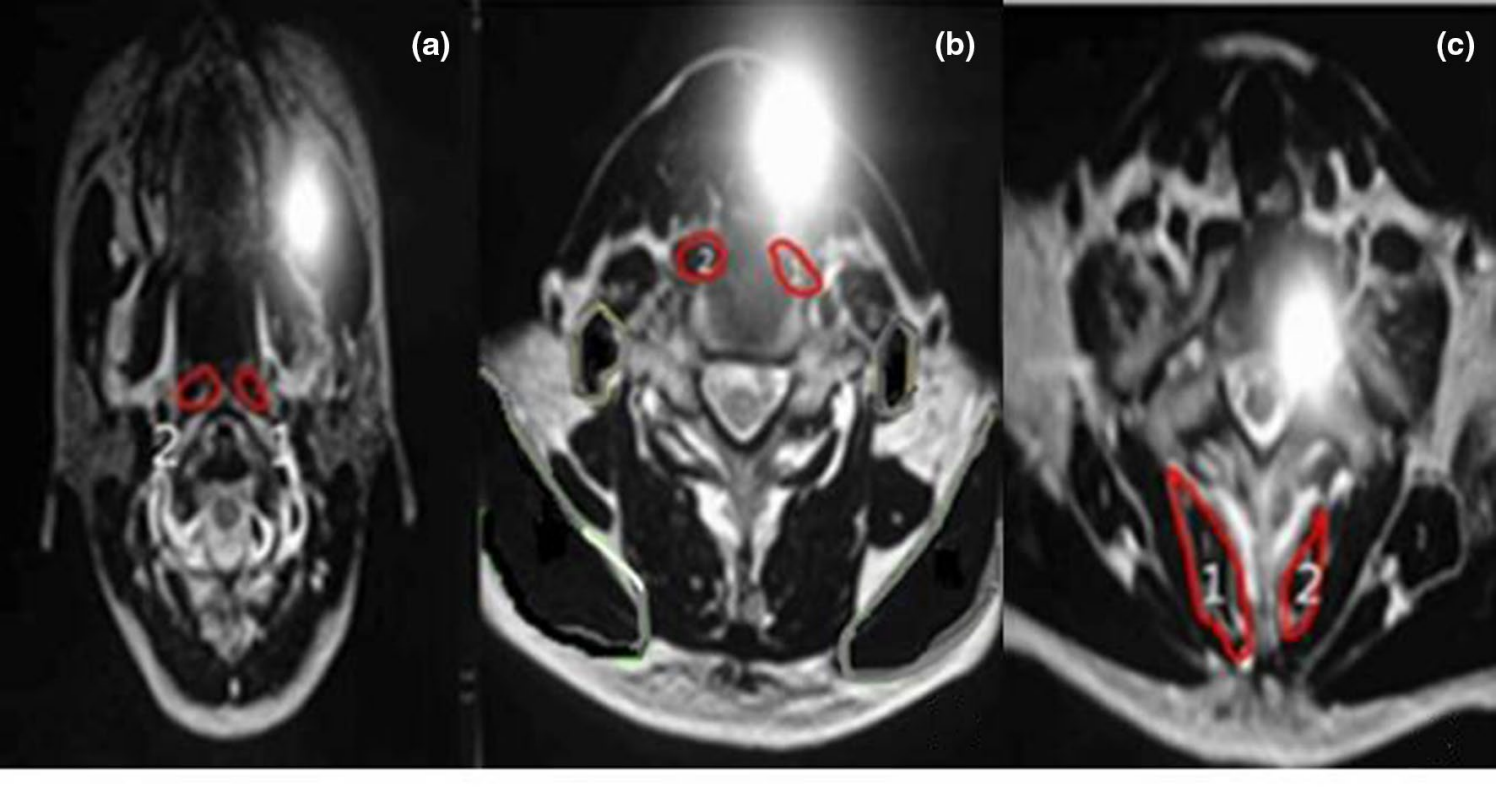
MRI (magnetic resonance imaging) imaging of the cross-sectional area of the perivertebral space. (a) Longus colli muscle; (b) Longus capitis muscle; (c) Multifidus muscle.
